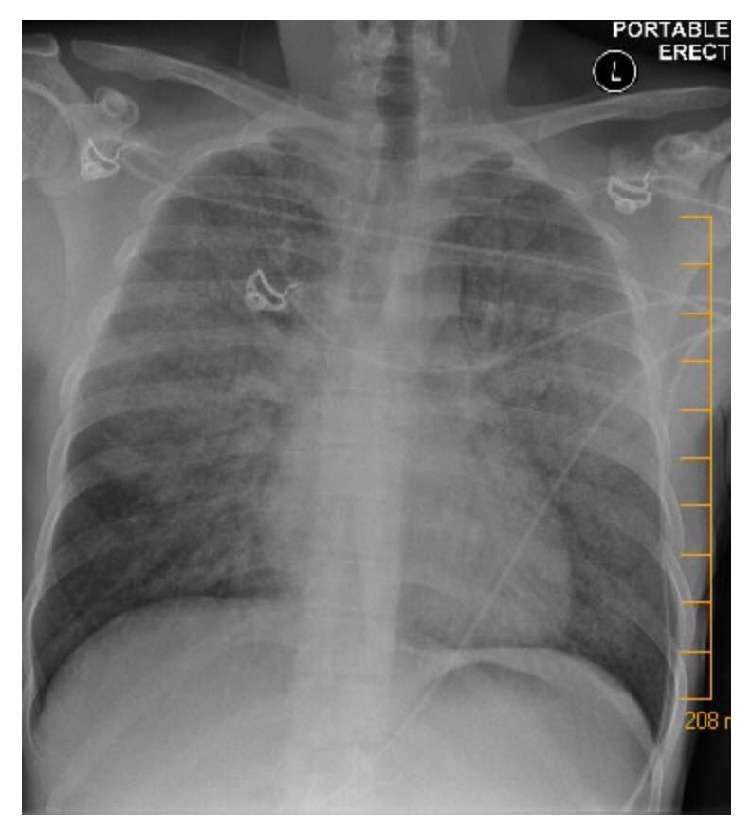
Anterior-posterior portable chest radiograph demonstrating bilateral pulmonary opacities, consistent with ARDS.
